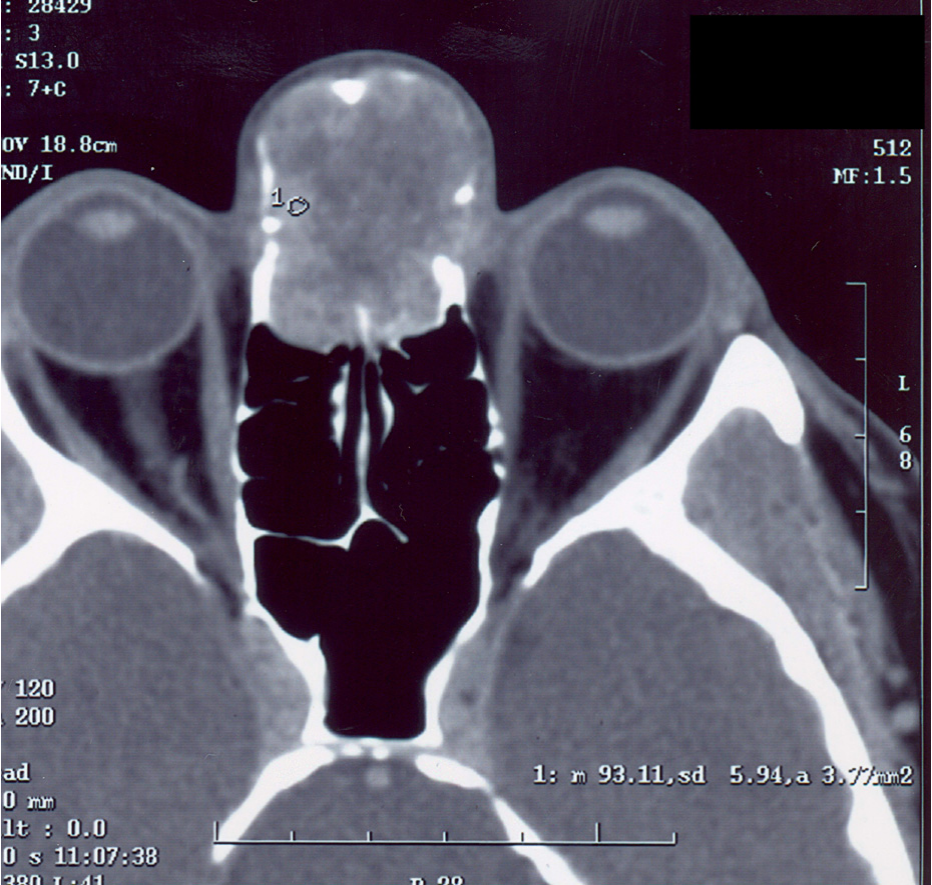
Axial CT scan. Well bordered soft-tissue tumormasses of a middle signal intensity with destruction of the nasal bones, usuration or even destruction of the medial orbital wall. Broadened nose root, prominence of the masses dorsally, up to the frontal ethmoid sinuses, rear ethmoides and sphenoidal sinus of normalairy character.
